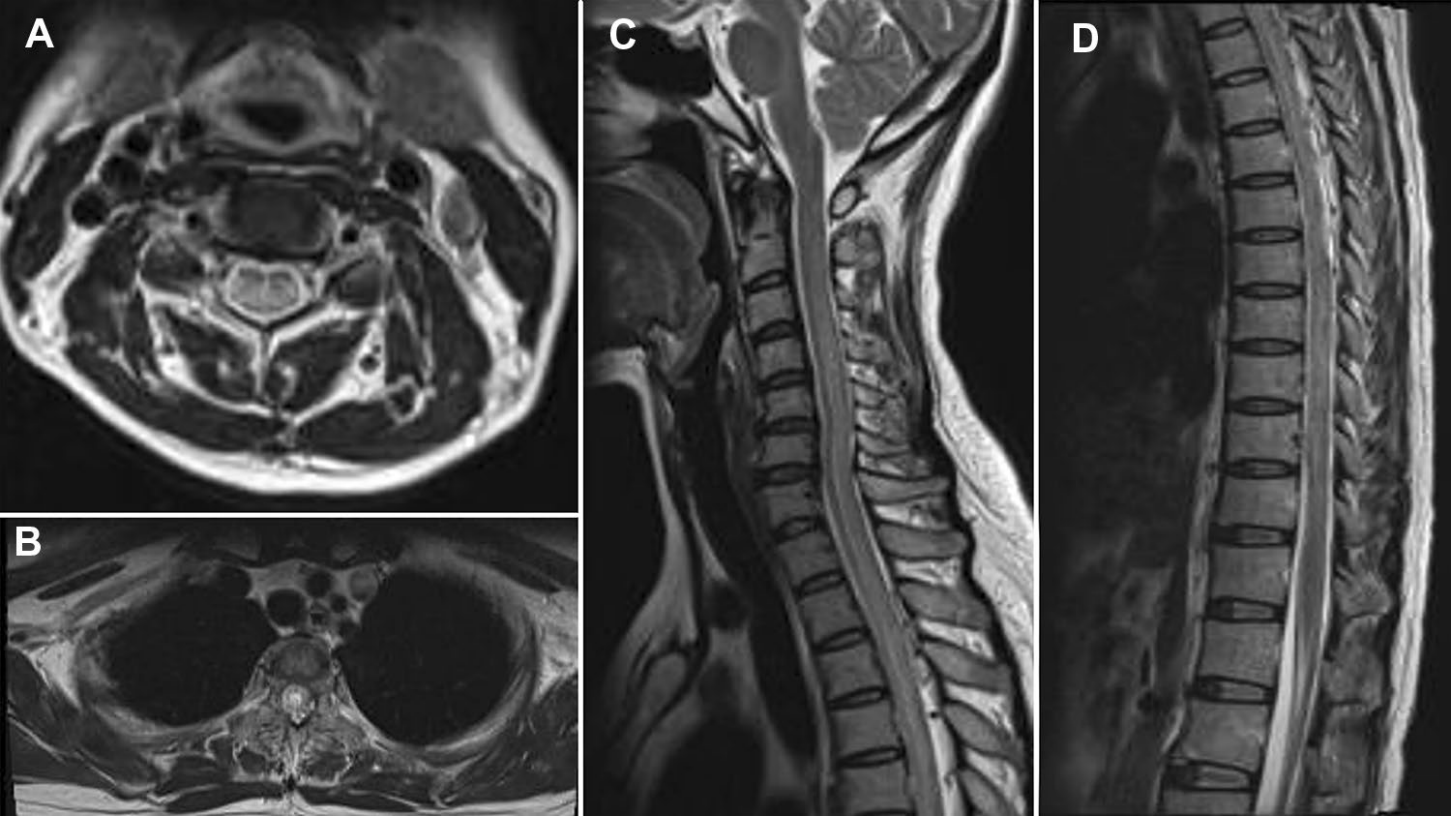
Spinal cord MRI showing strip and sheet-shaped long T2 signals in the horizontal spinal cord from the 2nd cervical vertebra to the 1st lumbar vertebrae, mainly involving the central part of the spinal cord (A, B, C, and D)
